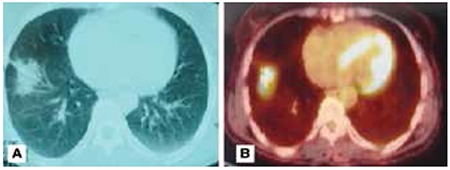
CT and PET-CT scans of a patient with focal lesionA) A focal lesion 3 cm in diameter with irregular borders radiating to the pleural surface in the right lower lobe. B) SUVmax of the lesion on PET/CT was 5.09. Trans-thoracic fine needle biopsy was insufficient for diagnosis, thus, it was surgically removed. The pathology of the lesion was reported as organizing peumonia
